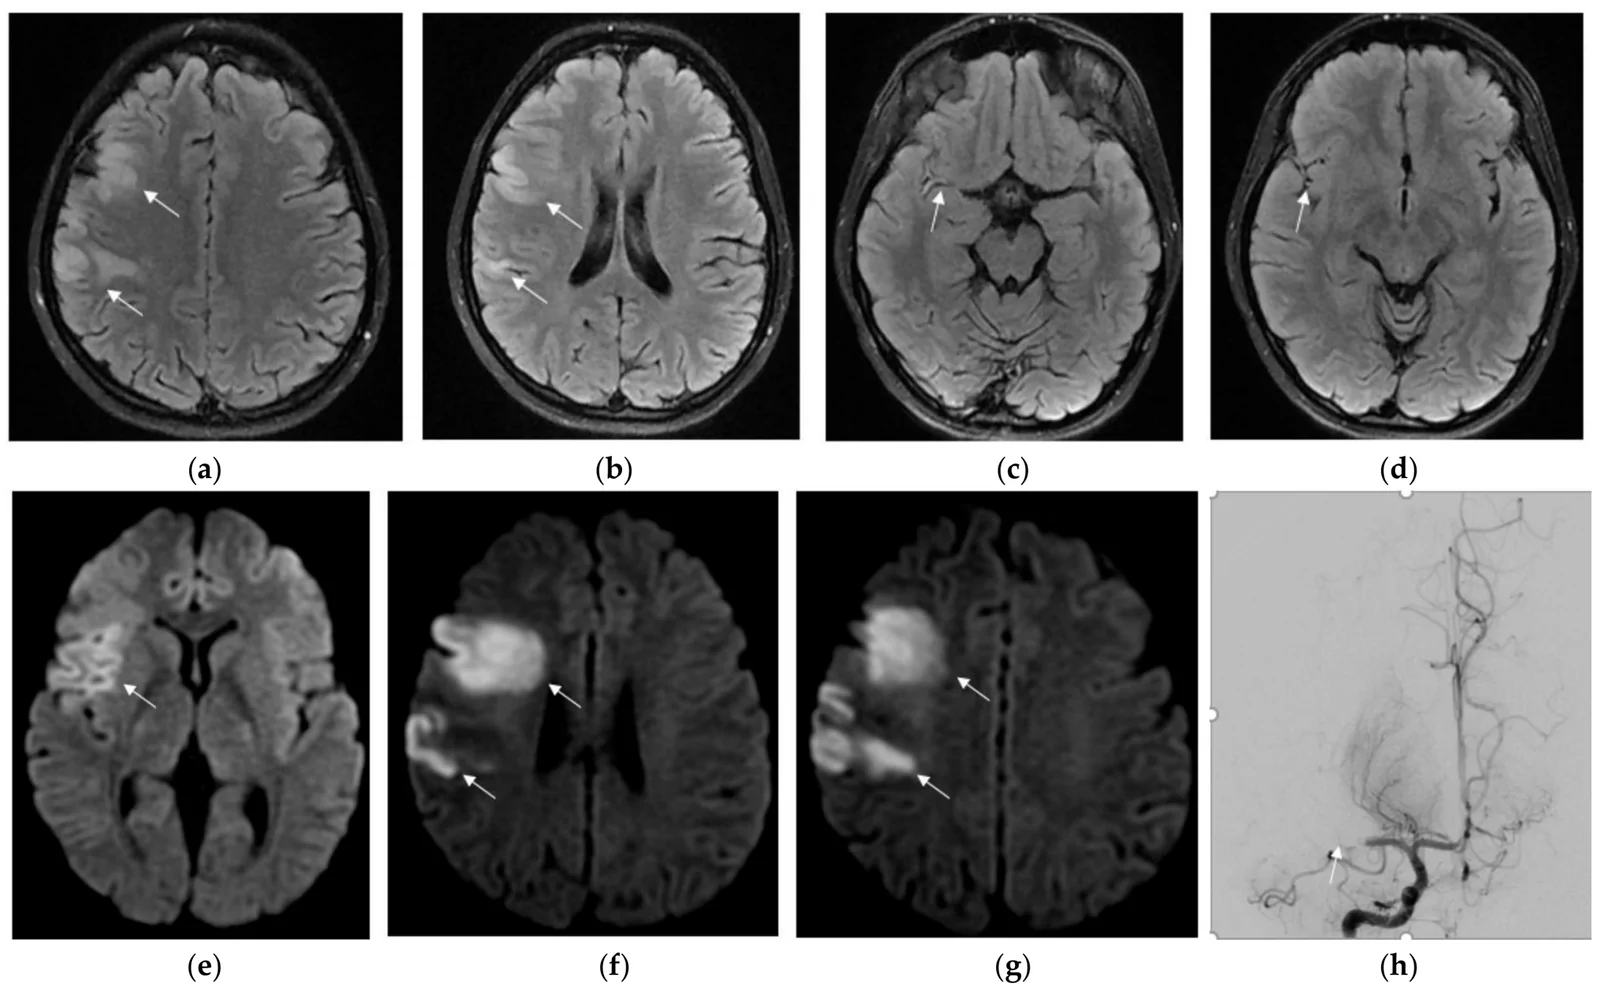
Neuroimaging findings in Case 3: a 16-year-old adolescent presenting with acute right MCA territory infarction in Takayasu arteritis. (a–d) Axial FLAIR images demonstrate hyperintense signal abnormalities in the right middle cerebral artery (MCA) territory consistent with acute ischemia. (e–g) Diffusion-weighted images demonstrate marked diffusion restriction in the right middle cerebral artery (MCA) territory consistent with acute infarction. (h) Digital subtraction angiography demonstrates distal occlusion of the right middle cerebral artery.
